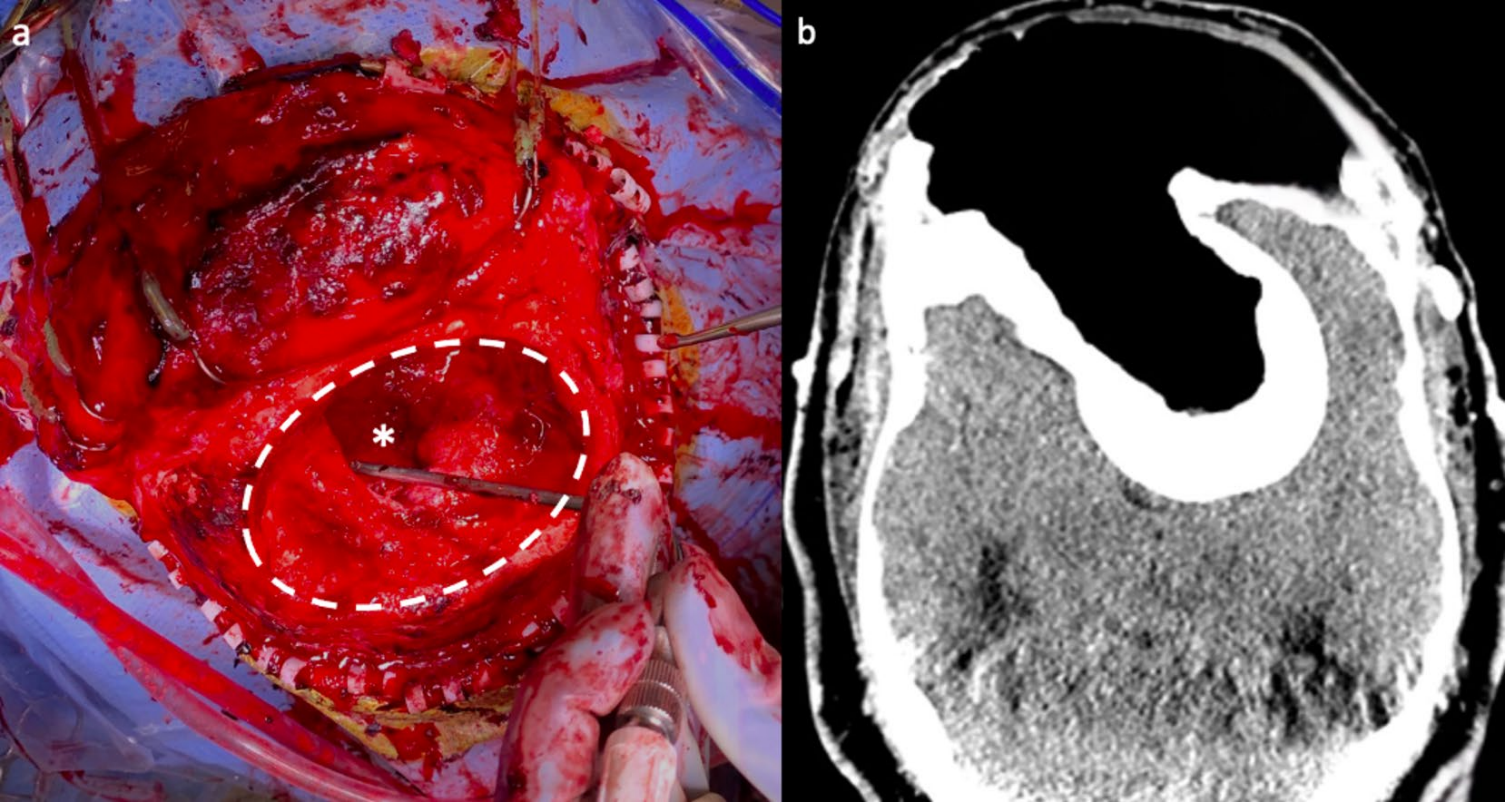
First surgery images. a Intraoperative picture showing the surgical cavity after the bifrontal craniectomy (dashed circle), drilling of the superficial lesion, and partial debulking of the intracranial mass (asterisk). b Axial CT scan after the first surgery demonstrated the removal of the superficial disease and intra-tumoral debulking of the pedunculated mass
